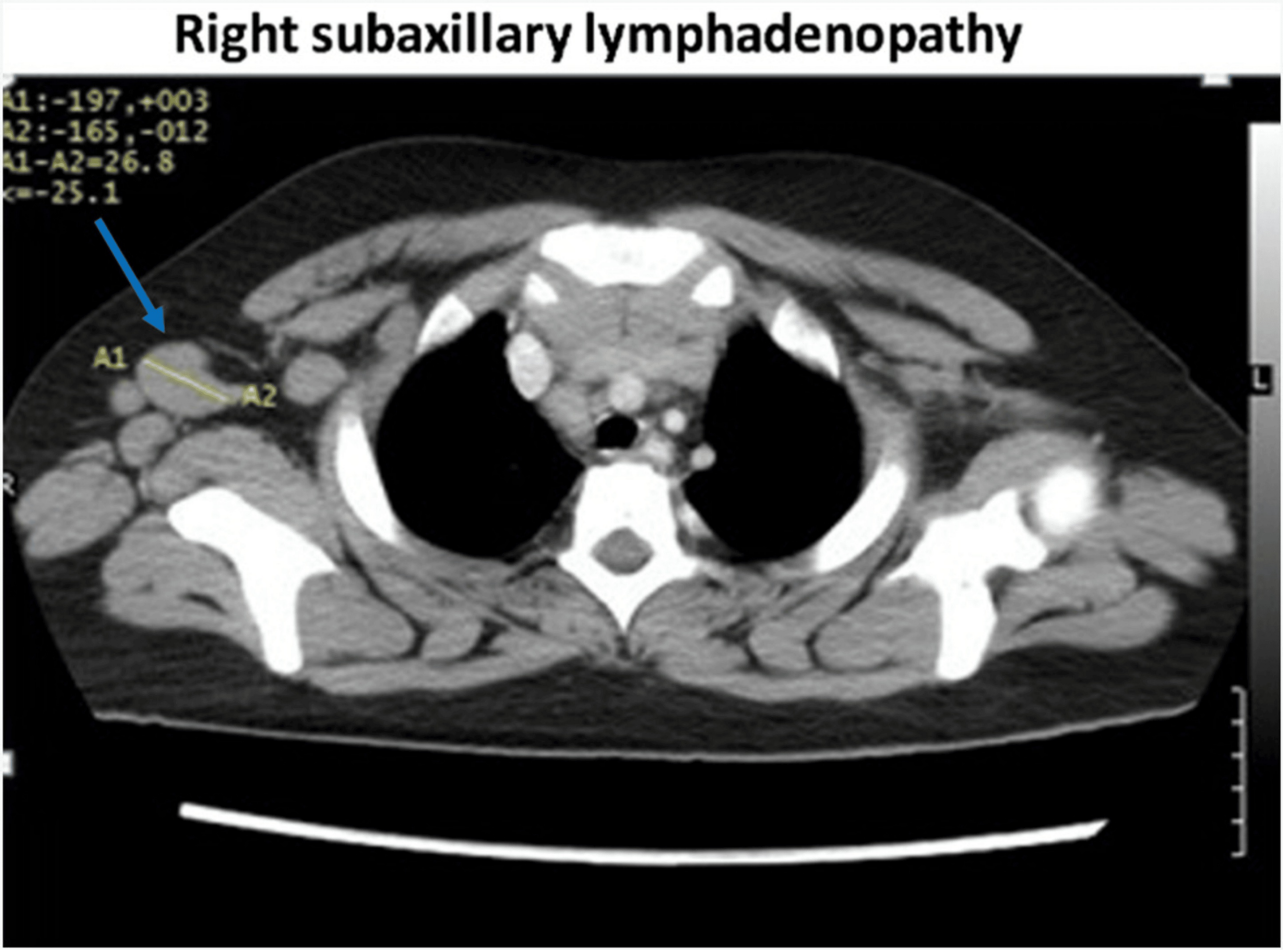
Abdominal CT scan showing the right subaxillary lymph node mass (blue arrow)
